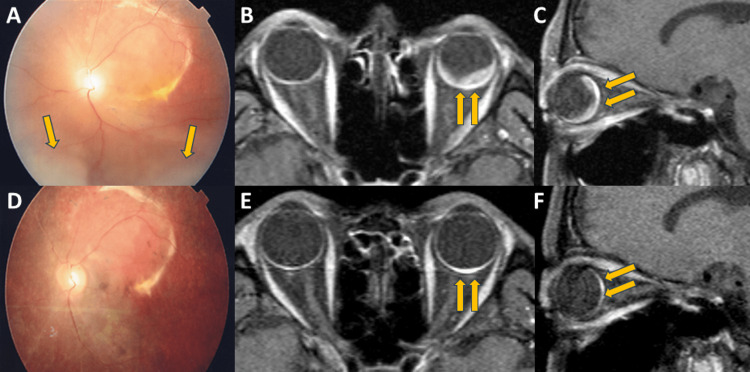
Color fundus photography (A, D) and contrast-enhanced MRI (B, C, E, F) of circumscribed choroidal hemangioma after proton beam therapy (A-C) One year after proton beam therapy. (D-F); Three years after proton beam therapy. (A, D) Color fundus photography revealed that the retinal detachment had improved one year after proton beam therapy, but some remained (arrows in A). It disappeared three years after proton beam therapy. (B, C, E, F) Contrast-enhanced MRI revealed gradual shrinkage of hemangioma over three years; however, a small lesion with contrast enhancement persisted (arrows in B, C, E, F).
